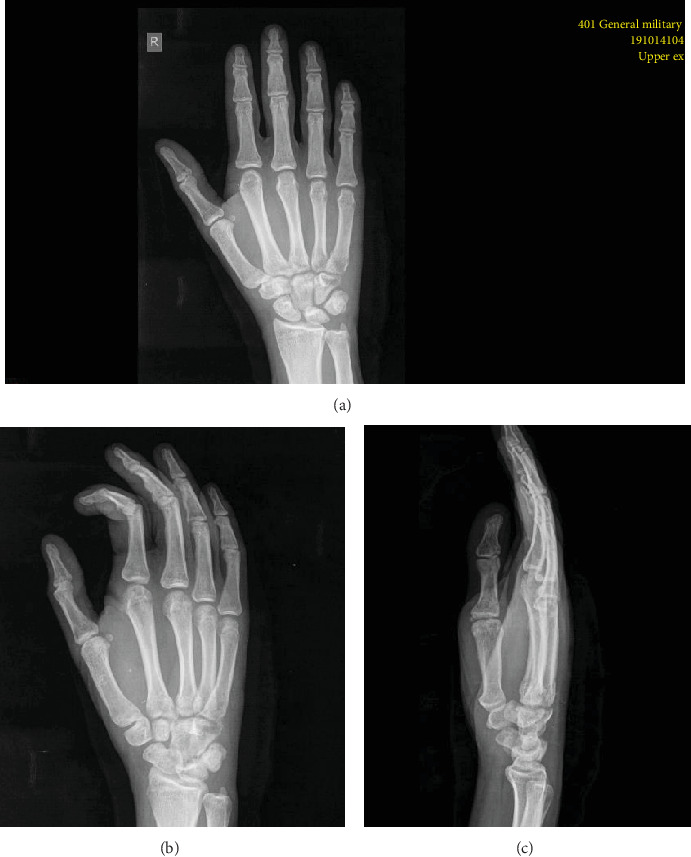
(a–c) Radiographs 6 weeks postoperatively after the K-wires' removal.
